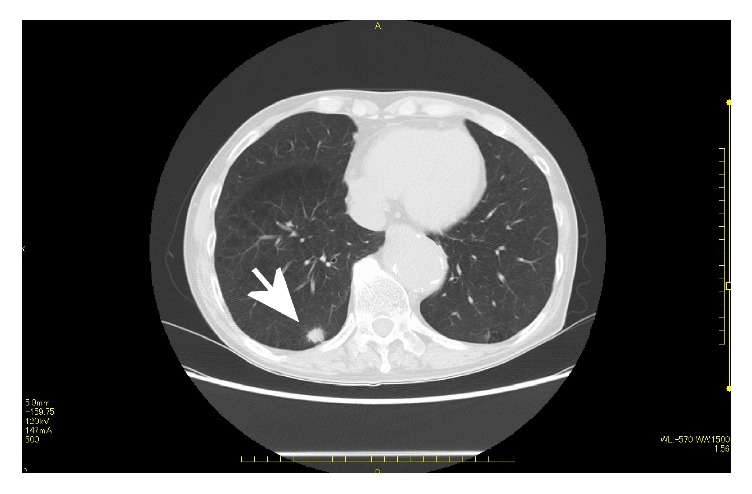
CT image showed right lung mass suspected for metastasis (arrow).
